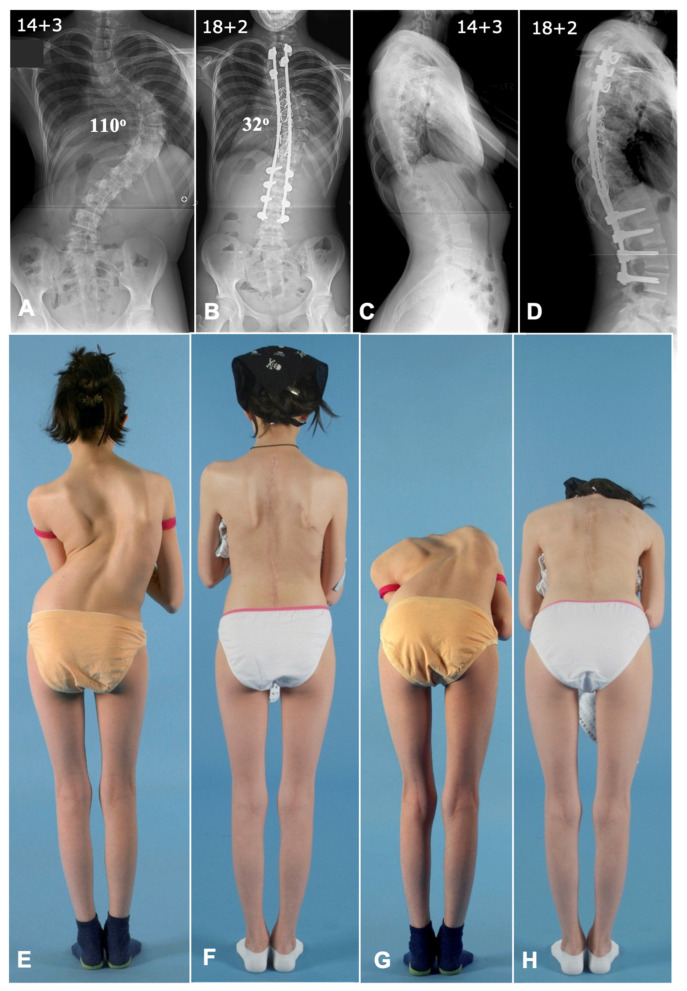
Deformity correction using a combined anterior and posterior approach in a severe rigid thoracic scoliosis case. (A,C) Preoperative antero-posterior and lateral radiographs showing a thoracic scoliosis with a Cobb angle of 110 degrees. (B,D) Postoperative radiographs showing good deformity correction with a Cobb angle of 32 degrees with well-maintained coronal and sagittal balance.(E,G) Preoperative clinical photographs showing a severe deformity and rib hump. (F,H) Postoperative clinical photographs showing a good correction of deformity and a well-maintained balance. Note the correction of the convex rib hump by anterior thoracoplasty.
